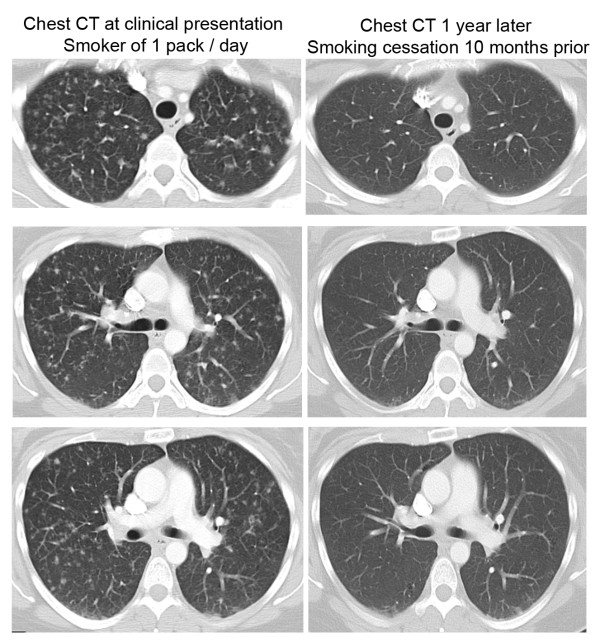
Radiographic improvement following smoking cessation. The chest CT images on the left side were performed in an active one pack/day 37 year old smoker with biopsy-proven PLCH. The representative chest CT images demonstrate diffuse nodular infiltrates in both upper and lower lung fields. The patient quit smoking 2 months after the first chest CT was performed. The representative chest CT images on the right side were performed one year after the first chest CT was obtained, and show considerable improvement in the nodular infiltrates following smoking cessation. The patient did not receive corticosteroid or other immunosuppressive therapy.
